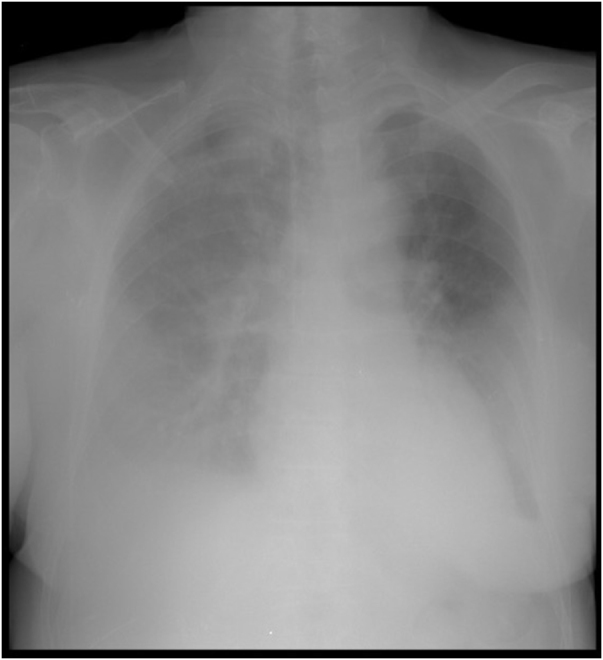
Postoperative X-ray after the excision of the right sternocostoclavicular joint including half of the manubrium, a segment of the clavicle, and the first rib via direct approach was performed. *Printed with permission; copyrights retained by Elise Lupon, MD, PhD.*
